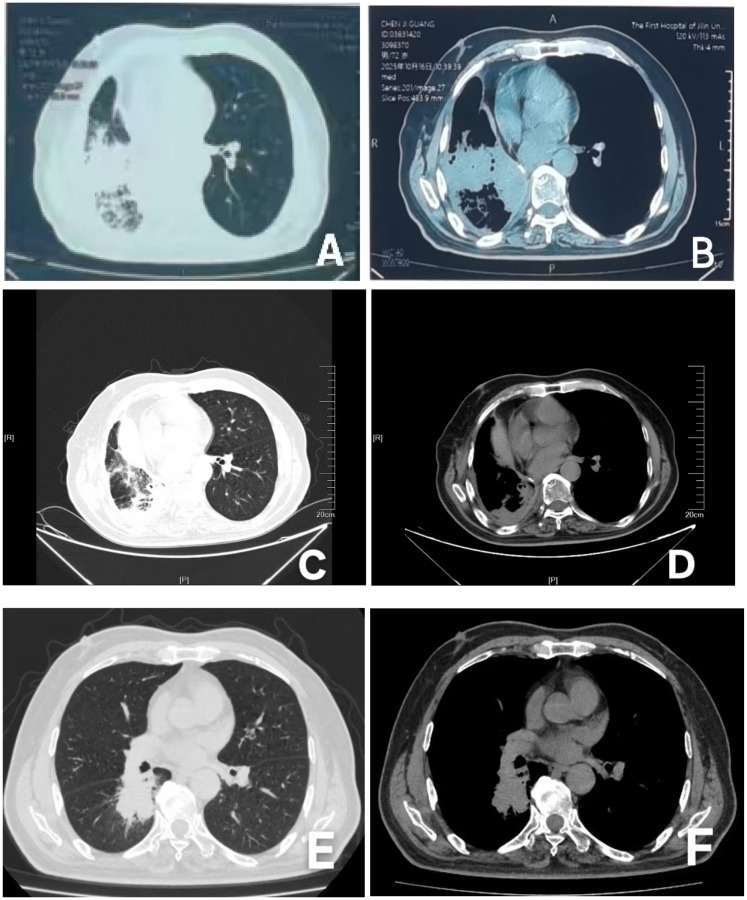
Chest CT imaging manifestations. ((A) Lung window at the initial diagnosis in 2026; mass in the right middle lobe. (B) Mediastinal window at initial diagnosis in 2026; mass in the right middle lobe. (C) Lung window at re-examination in 2026 after immunotherapy: postoperative changes in the right lung, multiple patchy high-density shadows in the residual right lung. (D) Mediastinal window at re-examination in 2026 after immunotherapy: postoperative changes in the right lung, multiple patchy high-density shadows in the residual right lung. (E) Lung window at the initial diagnosis in 2022; mass across the oblique fissure involving the posterior segment of the right upper lobe and the dorsal segment of the right lower lobe. (F) Mediastinal window at initial diagnosis in 2022; mass across the oblique fissure involving the posterior segment of the right upper lobe and the dorsal segment of the right lower lobe).
